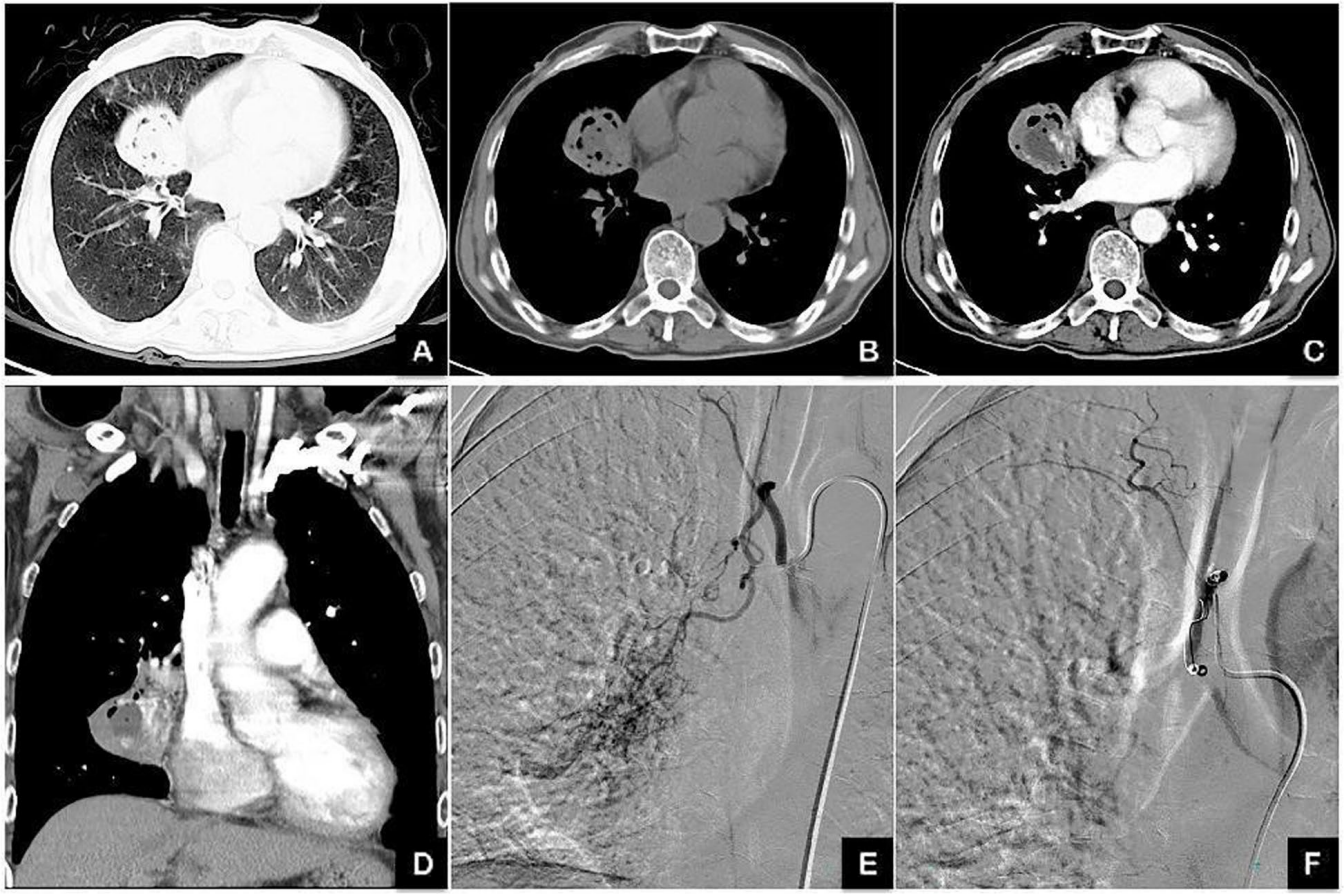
High resolution chest CT (HRCT) and digital subtraction angiogram before and after the BAE in case #1. A 62-year-old male patient had history of intermittent hemoptysis for 30 years, became worse for one month with approximately 150 mL of blood each time. A-D: High resolution chest CT (HRCT) images showing reduced volume and uneven density in the right middle lobe as well as cavities and necrosis. E: Digital subtraction angiogram after selective catheterization of the right bronchial artery and super-selective catheterization with a microcatheter, showing hypertrophied artery and hyper-vascularity in the right middle lobe; F: Digital subtraction angiogram after embolization, demonstrating no residual flow to the lesion
